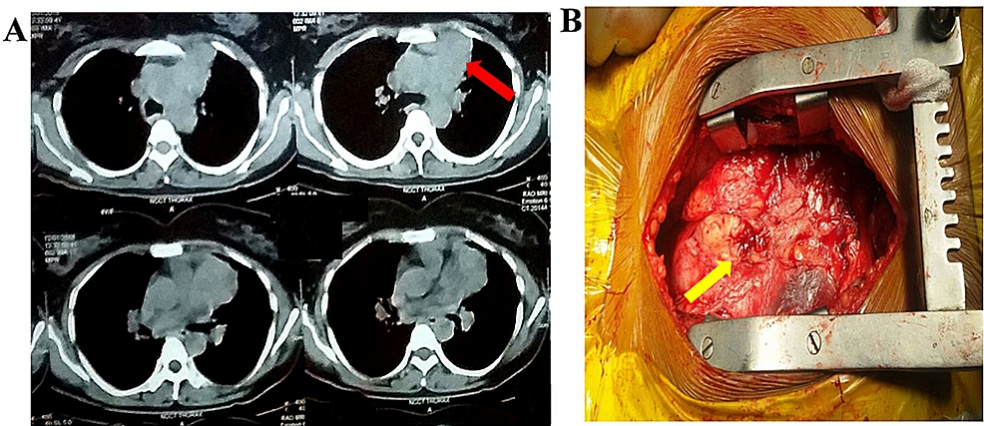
(A) Contrast-enhanced CT scan; (B) intraoperative picture. Red and yellow arrows represent thymic carcinoma in radiological and intraoperative figures, respectively. CT: computed tomography
